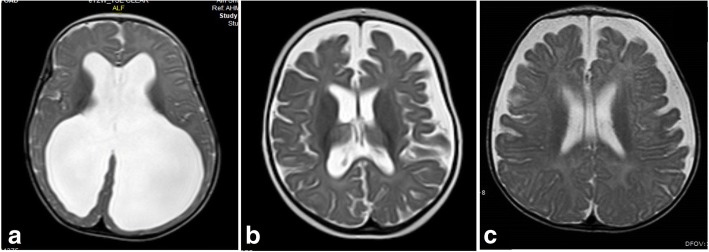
Axial T2-WI in different patients with ventriculomegaly a MRI of a 7-month-old girl with hydrocephalus associated with septo-optic dysplasia showing ventricular dilatation with effacement of extra-axial CSF spaces. b MRI of an 8-month-old boy with delayed development and microcephaly showing ventricular dilatation ex-vacuo; both lateral ventricles and extra-axial CSF spaces are dilated. c MRI of a 5-month-old girl with macrocephaly with normal development showing benign enlargement of subarachnoid spaces
